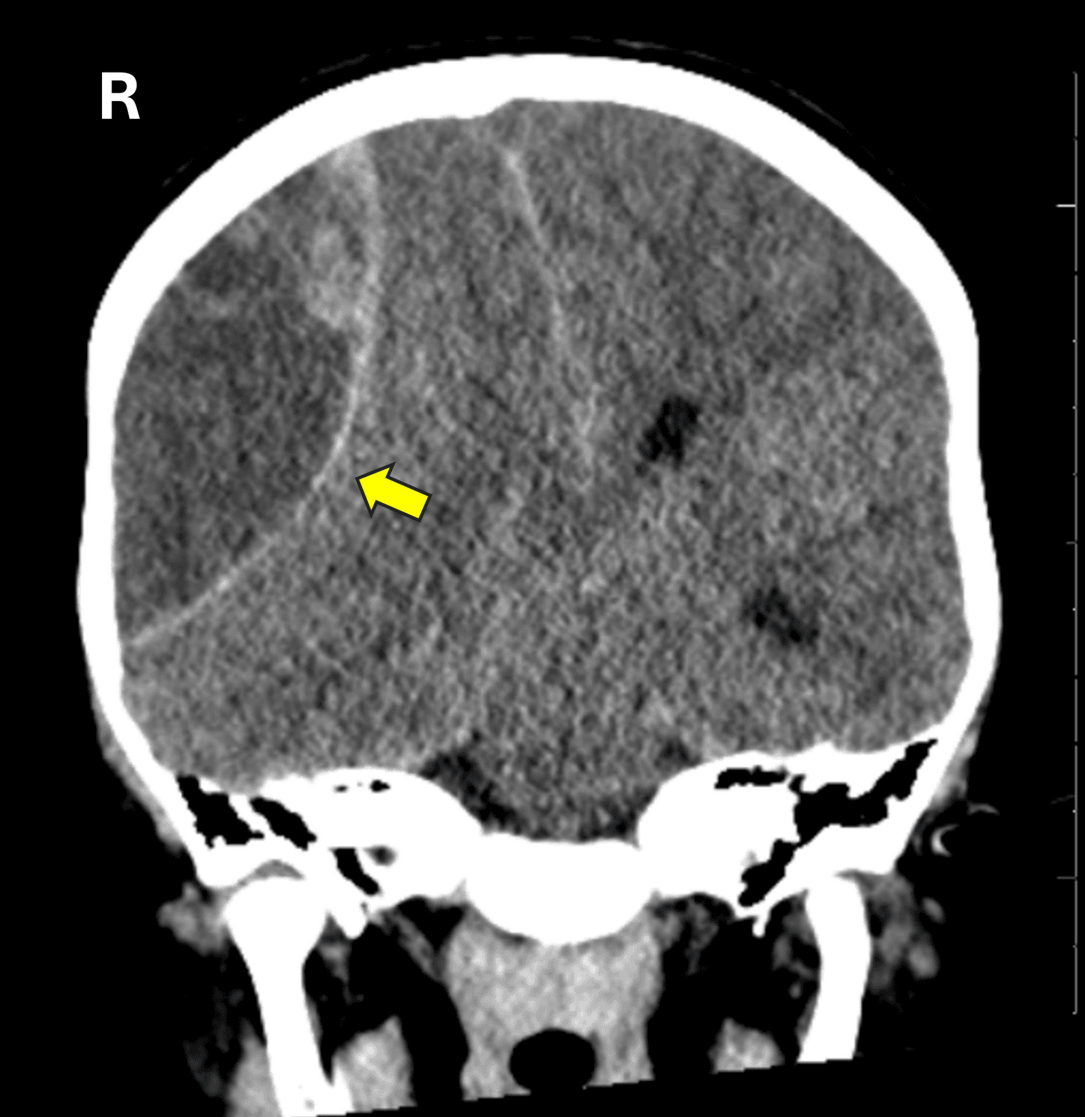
Non-contrast brain CT scan of case 2, coronal view. The images show a sizable right-sided extra-axial lentiform-shaped area of mixed density, with hyperdensity observed in the dependent portion, indicative of an extradural hematoma. There is a noticeable mass effect and midline shift.
